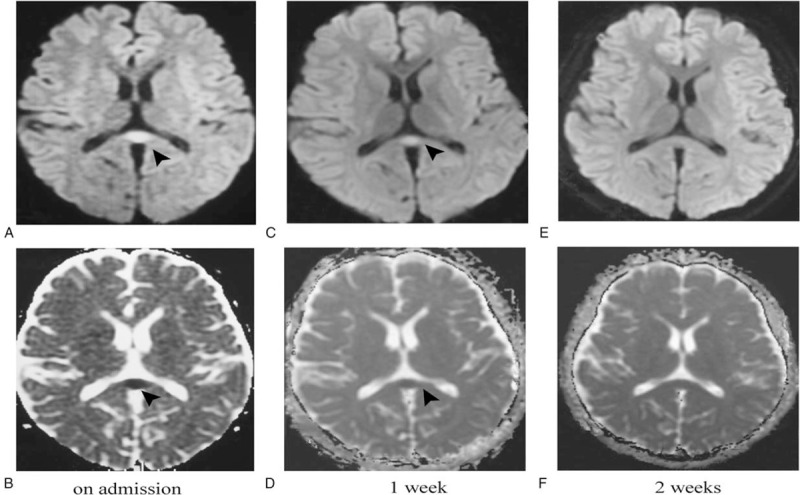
Brain magnetic resonance imaging (MRI) changes of the lesion in the splenium of corpus callosum (SCC) among the initial images and at 1-week and 2-weeks of follow-up. Initial MRI images show an ovoid isolated lesion in the SCC (arrow), exhibiting hyperintensity on diffusion-weighted imaging (DWI) (A) and hypointensity on apparent diffusion coefficient (ADC) (B). The lesion signal (arrow) weakened on a review MRI a week later (C, D), and completely disappeared on the follow-up MRI two weeks later (E, F).
